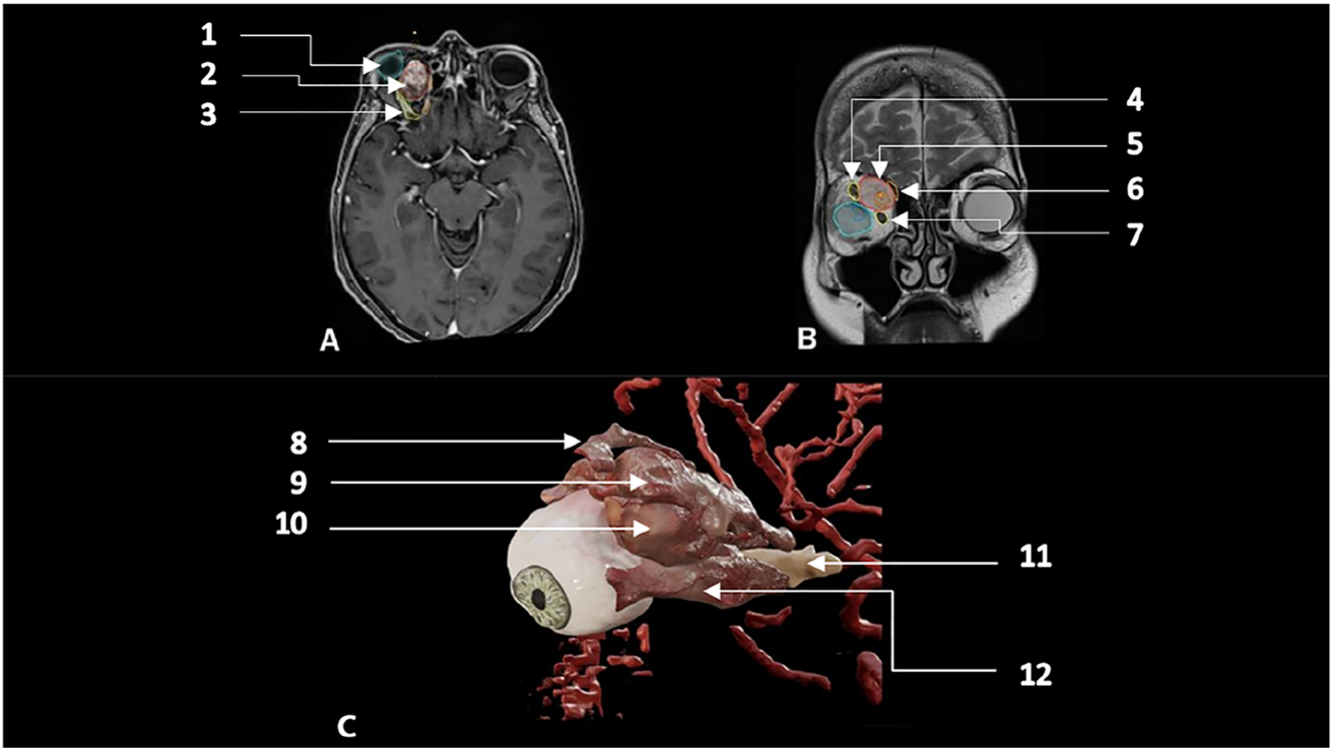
Preoperative 3D-modeling. Relevant surgical structures are displayed. (A) Axial- and (B) coronal-T1-weighted, contrast enhanced sequence with automated and manual segmented relevant surgical structures. (1) Globe, (2) tumor mass, (3) *M. rectus* superior, (4) *M. rectus* superior, (5) tumor, (6) *M. obliquus* superior, (7) *M. rectus* medialis, (C) 3D volumetric model of segmented structure on the level of orbit. (8) *M. obliquus* superior, (9) *M. rectus* superior, (10) tumor, (11) optic nerve and (12) *M. rectus* medialis.
